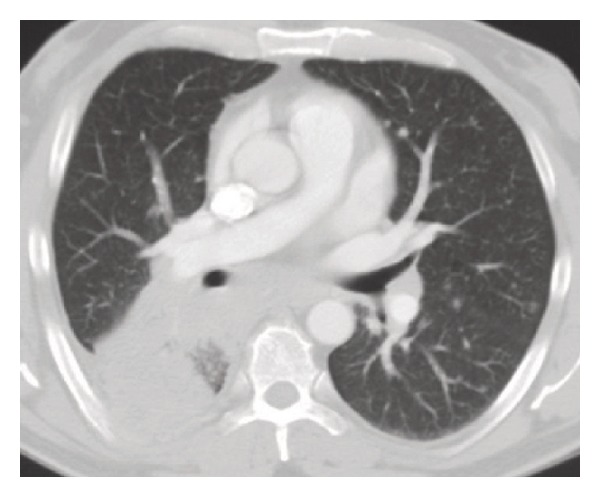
CT scan chest at presentation showing areas of consolidation in the right lower lobe. Also note the small right pleural effusion.
